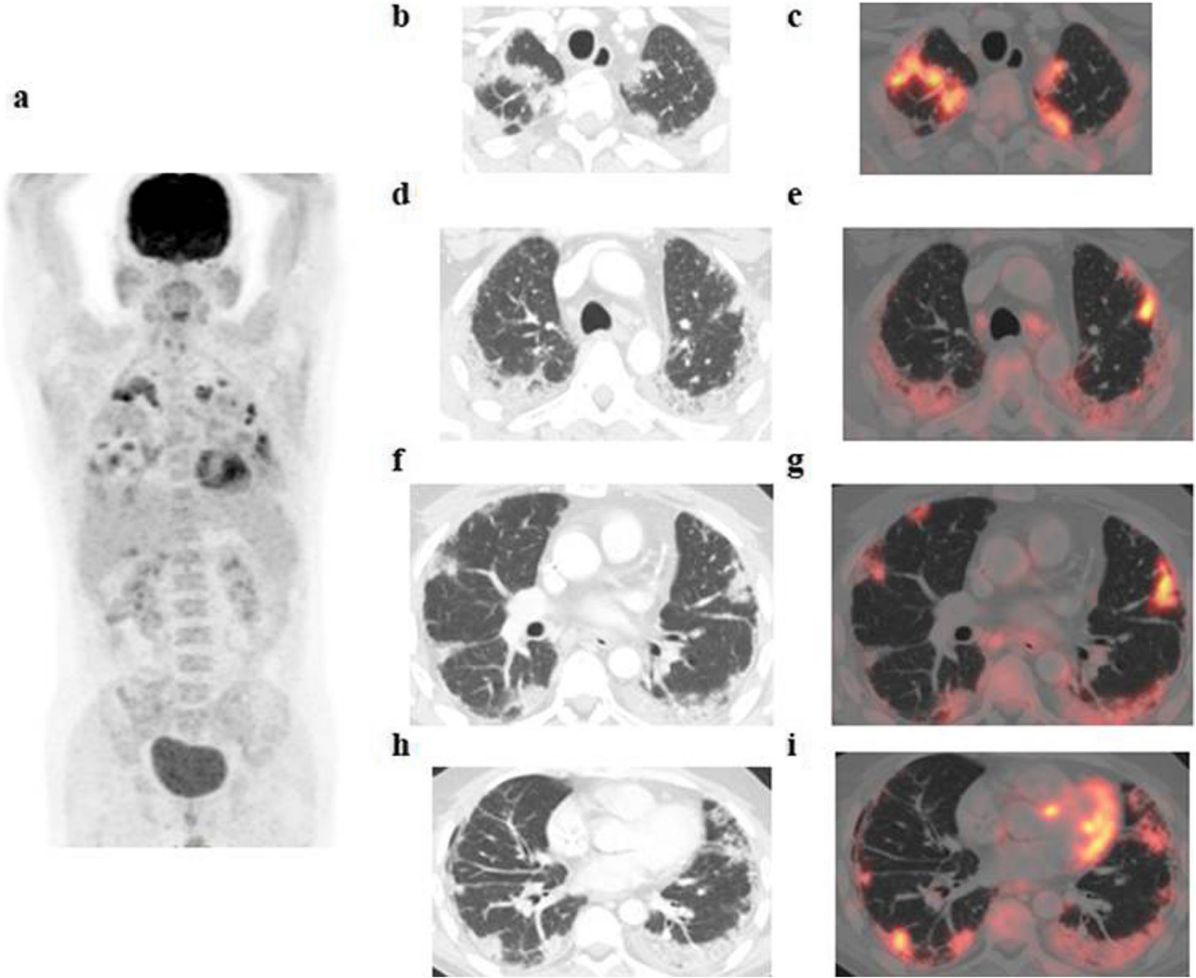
A 47-year-old male patient, with a history of left testicular seminoma, underwent left orchidectomy and received CTH, referred for follow-up PET/CT examination. The whole-body MIP image (a) shows bilateral multiple pulmonary metabolically active areas. Axial lung window CT images (b, d, f, h) revealed multiple bilateral subpleural variable-sized consolidations and ground-glass opacities with crazy-paving appearance that show variable FDG avidity on corresponding fused PET/CT images (c, e, g, i) achieving up to 10.9 SUVmax
